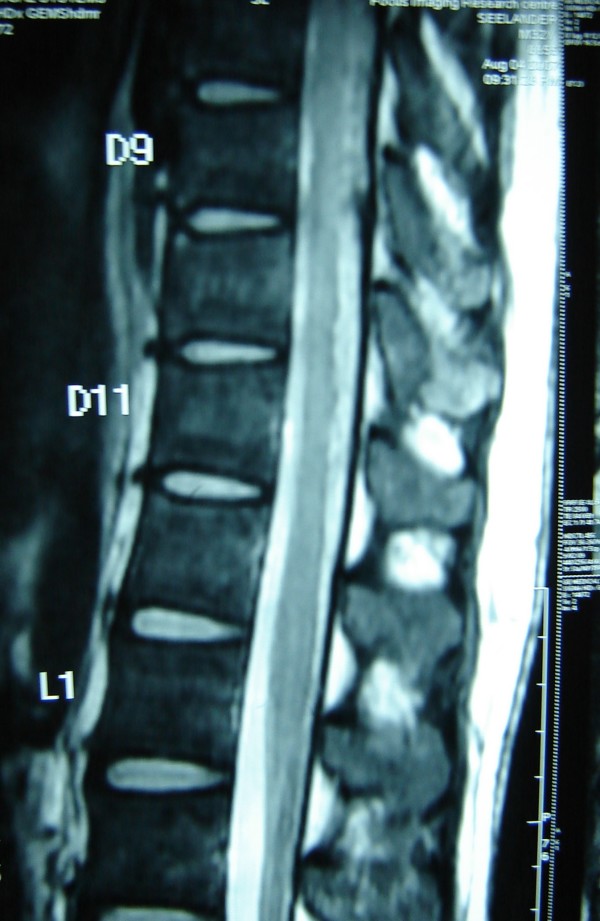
MRI of spine showing myelitis.
